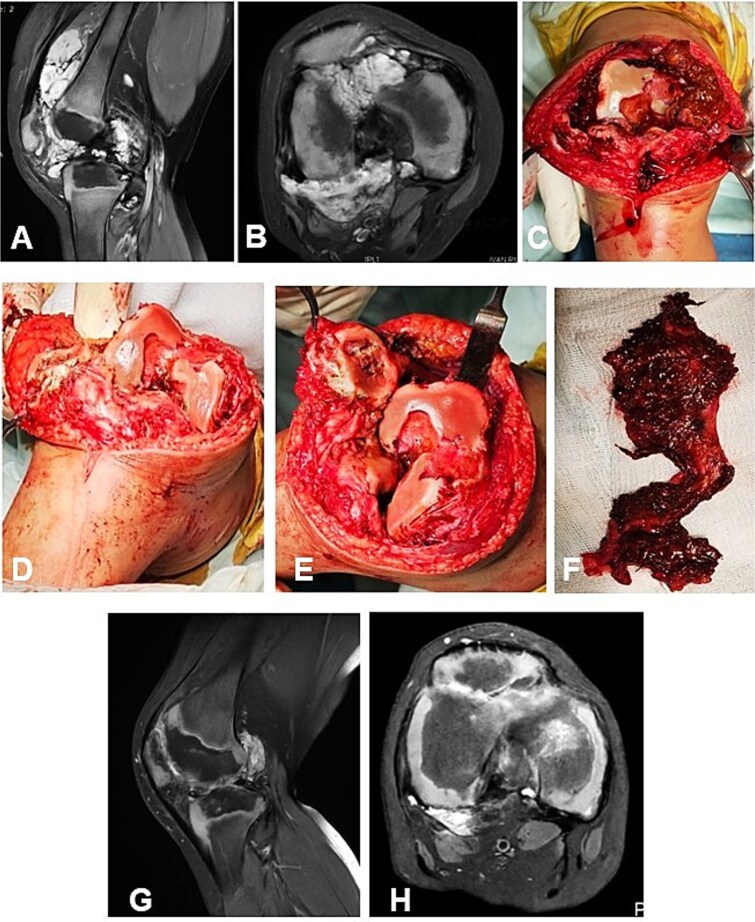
Case 1. (A and B) Preoperative T1 sagittal and axial MRI sequences with PD FS. (C and F) Macroscopic appearance of the pathological synovium before and after its excision. (D and E) The knee joint following synovectomy, demonstrating intact menisci and cruciate ligaments. Chondral lesions correspond to Outerbridge grade IV. (G and H) Postoperative 24 m. Sag and Ax MRI PD FS. No evidence of recurrence of the resected neoplasm was detected. Residual TGCT involvement persists within the lateral posterior femoral recesses. PD FS, proton density weighting with fat suppression; TSE, turbo spin echo.
